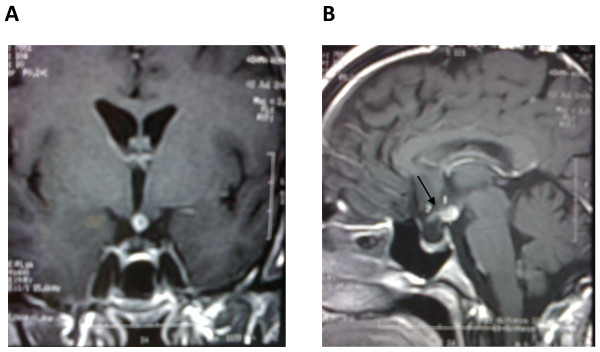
MRI of the brain coronal (A) and sagittal (B) T1-weighted images with contrast enhancement, performed at the first presentation showing the suprasellar mass(1) with thickening of pituitary stalk (2).
